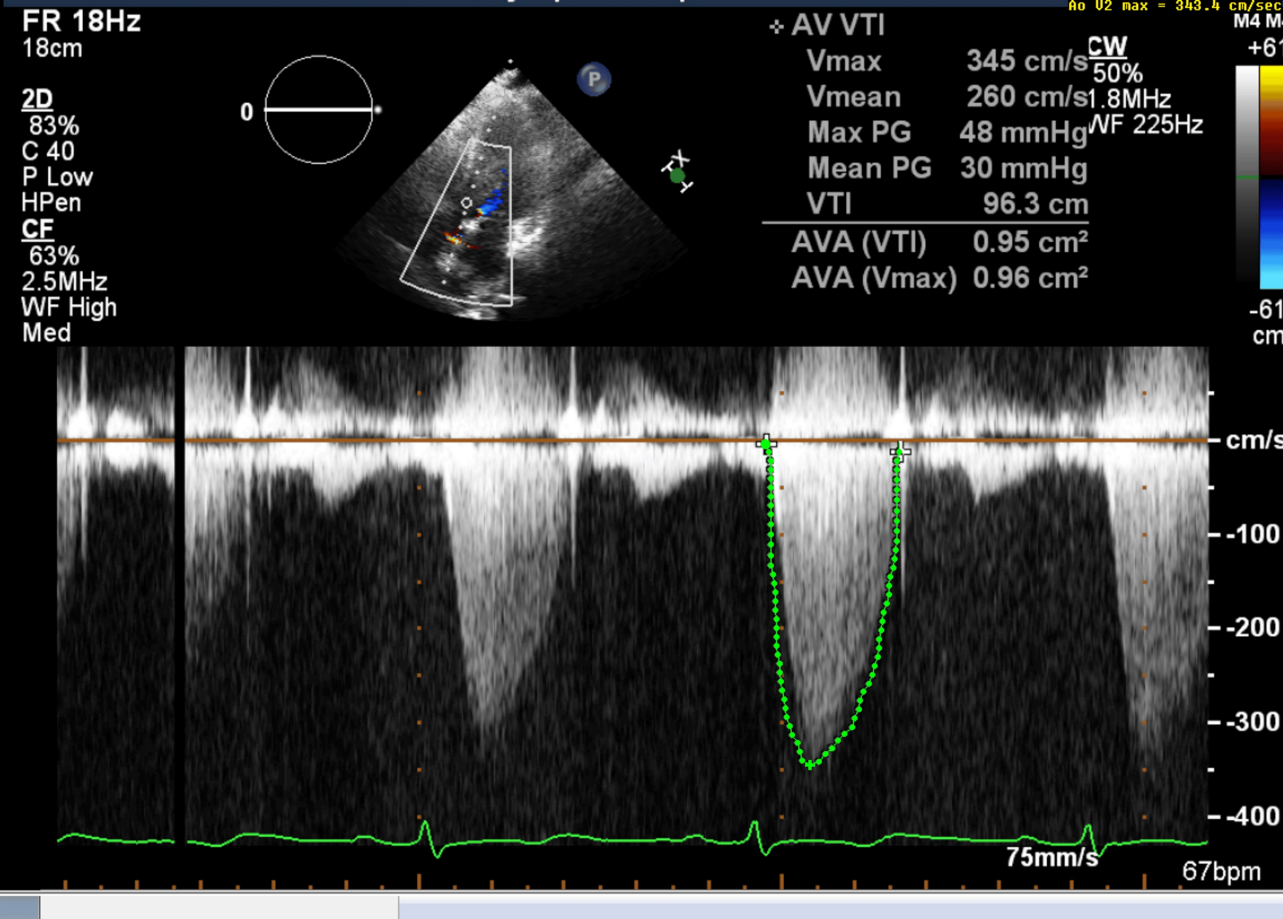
Doppler echo showing LVOT obstruction LVOT: left ventricular outflow tract
